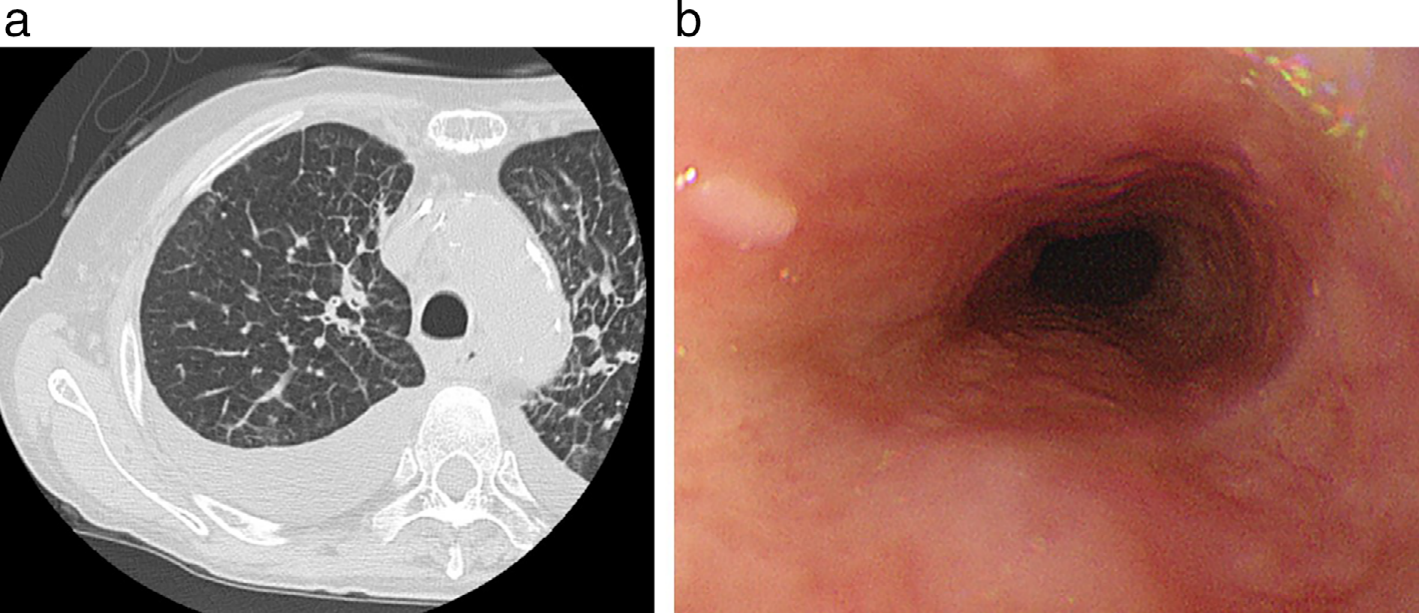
(a) Computed tomography (CT) showed the cancerous lymphangiopathy had improved, and (b) upper gastrointestinal endoscopy showed the esophageal stricture had improved
